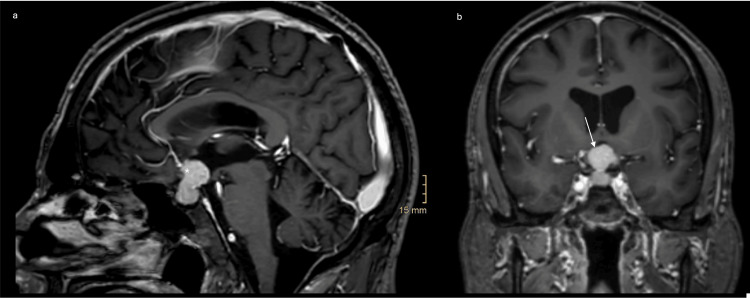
Preoperative cranial MRI with contrast medium Preoperative (a) sagittal and (b) coronal T1-weighted MRI showing a contrast-enhancing mass in the sella turcica with progressive suprasellar extension (asterisk/arrow) compared to the previous image.
